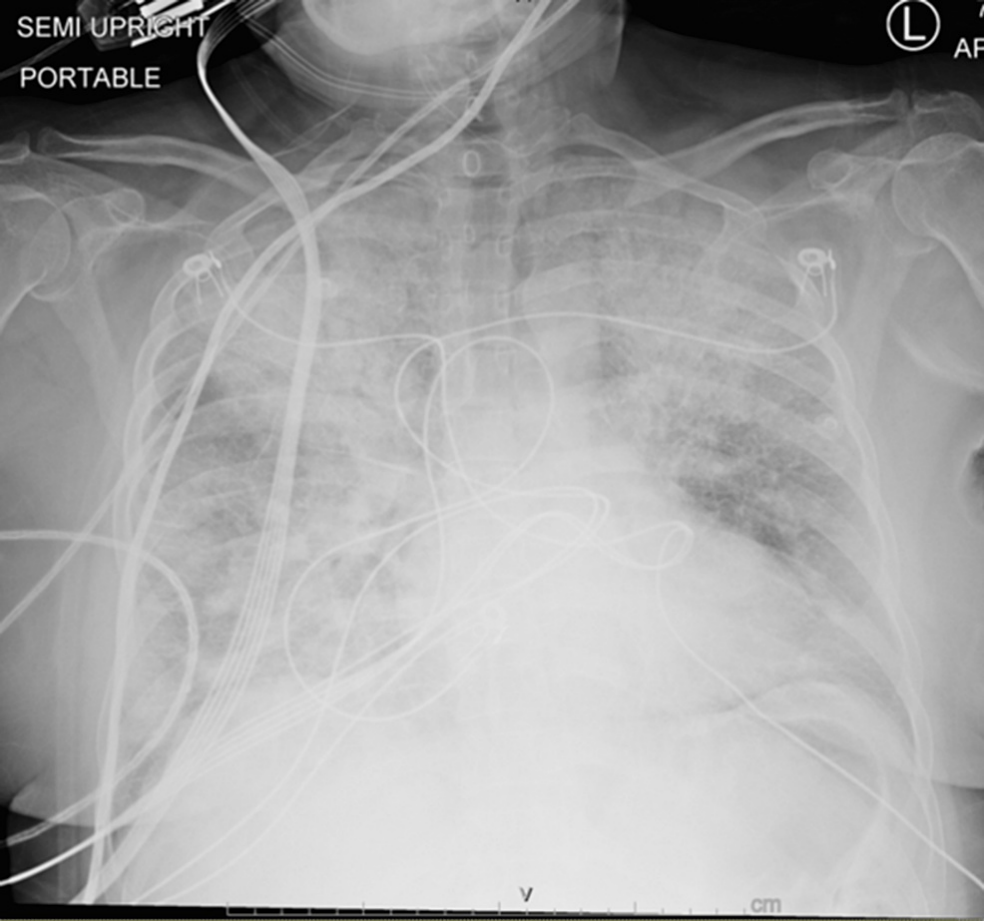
Chest X-ray: bilateral interstitial opacities
